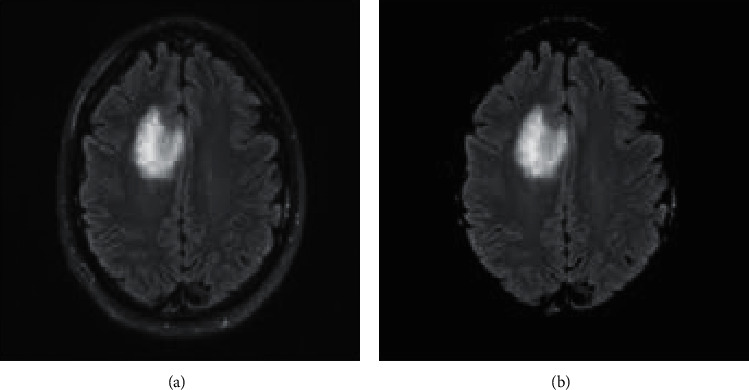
MRI image (a) and its skull stripped image (b).
